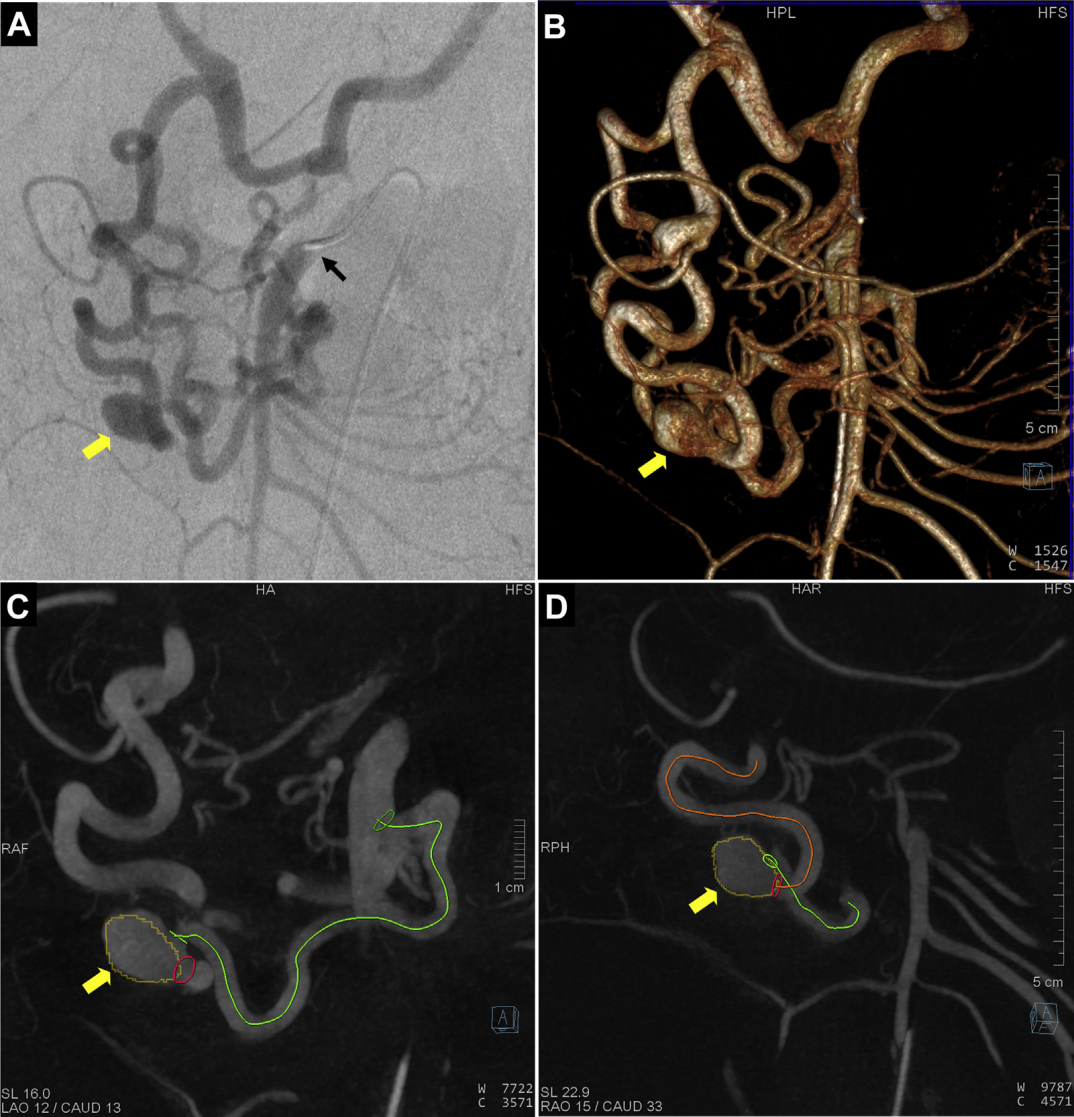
A, Selective arteriogram of superior mesenteric artery (SMA; *black arrow*) with an aneurysm involving the anterior pancreaticoduodenal arcade (*yellow arrow*). B, Volume rendered reconstruction of cone-beam computed tomography angiography (CBCTA) showing the pancreaticoduodenal arcade, three-dimensional (3D) aneurysm morphology, and inflow and outflow vessels. C and D, 3D planning of aneurysm for embolization with ostia of inflow vessels (*green centerline*) from SMA and outflow vessels (*orange centerline*) electronically marked in two oblique views.
